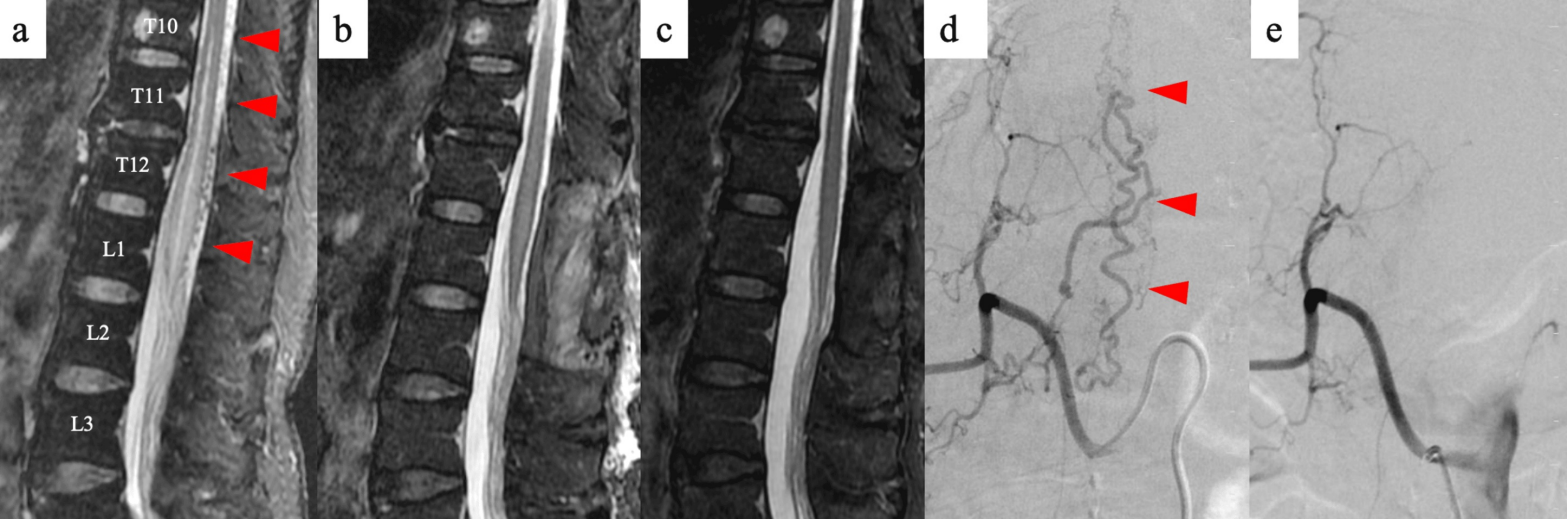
Imaging findings before and after surgery in Case 1 (a) T2-weighted MRI scan before surgery showing a high signal area in the spinal cord and dilated vessel on the dorsal surface of the spinal cord; (b) Postoperative T2-weighted MRI does not show a high signal area in the spinal cord and dilated vessel on the dorsal surface of the spinal cord; (c) T2-weighted MRI scan at three years after surgery, during the follow-up evaluation, does not show a high signal area in the spinal cord and dilated vessel on the dorsal surface of the spinal cord; (d) Angiography before surgery shows an sdAVF with L1-2 right root artery as the feeding vessel; (e) Angiography after surgery does not show an sdAVF with L1-2 right root artery as the feeding vessel. The red arrowheads indicate findings of a spinal dural arteriovenous fistula.
